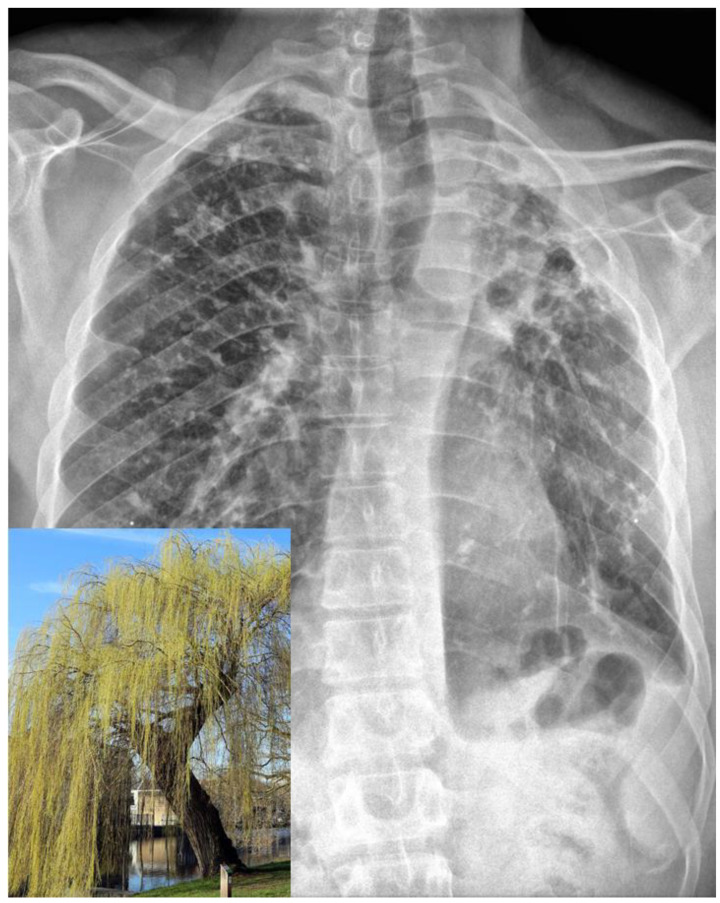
The patient’s chest X-ray exhibits the classic “weeping willow” sign within the left hemithorax, which is a distinctive feature of pulmonary scarring induced by tuberculosis. This sign results from a combination of apical contraction, bronchial drooping, and fibrotic bands that extend down to the diaphragm, creating a resemblance to a weeping willow tree. Inlet, bottom left: weeping willow tree, for comparison. Inlet image courtesy of Sailko, CC BY 3.0, via Wikimedia Commons.
